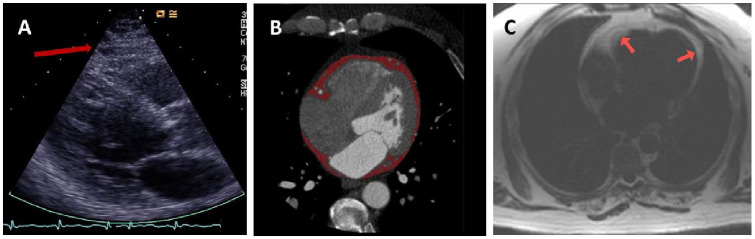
(a) Imaging of EAT by echocardiography. (b) Cardiac computed tomography^
